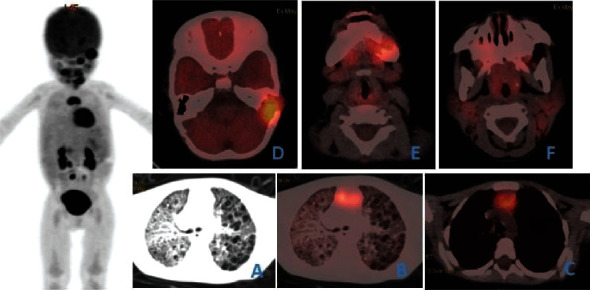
Female, 1 year old, MS-LCH (bone marrow + lung + lymph nodes). (a, b) Extensive cystic low-density shadow in both lungs without significant ^18^F-FDG uptake. (c) Enlarged lymph nodes in the anterior mediastinum increased ^18^F-FDG uptake and SUVmax of 5.7. (d, e) Penetrating bone destruction and local soft tissue density shadow in the left temporal bone and mandible increased ^18^F-FDG uptake and SUVmax of 6.4. (f) Bilateral cervical multiple enlarged lymph nodes on CT, with the larger one of 15 × 11mm, but without significant ^18^F-FDG uptake. LCH infiltration was excluded via pathology.
